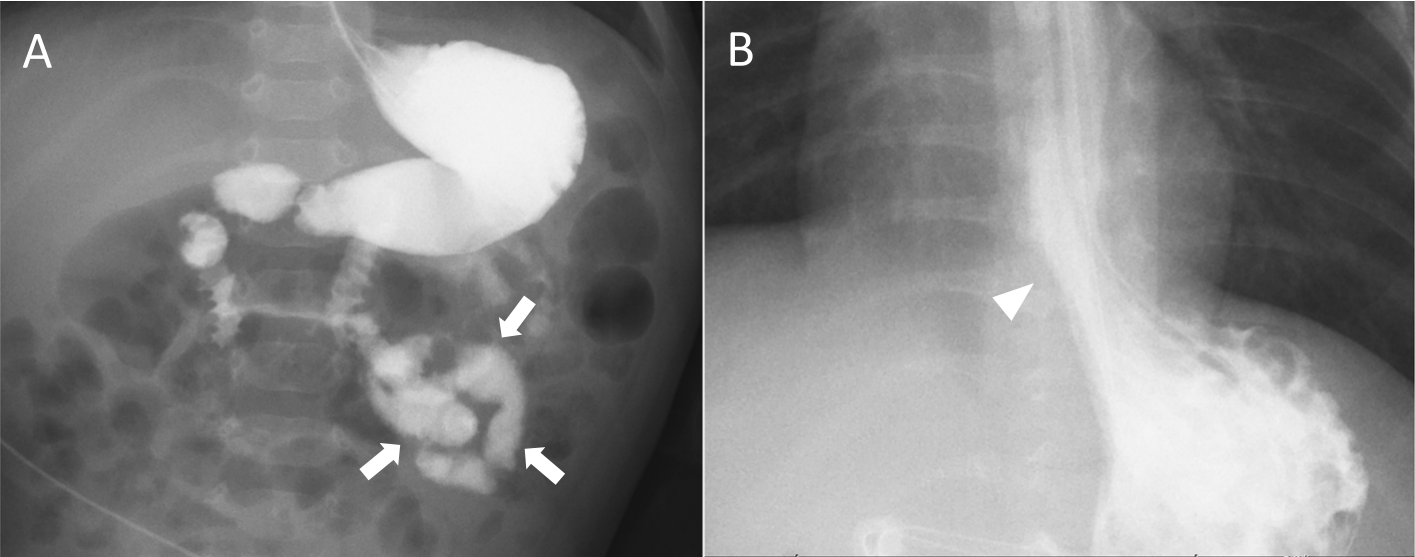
Gastrointestinal phenotypes associated with Pierson syndrome. a An upper gastrointestinal contrast study depicting the intestinal malrotation. Arrows indicate the “corkscrew sign” of the proximal jejunum. b A radiographic contrast image depicting the gatroesophageal reflux (arrow head)
